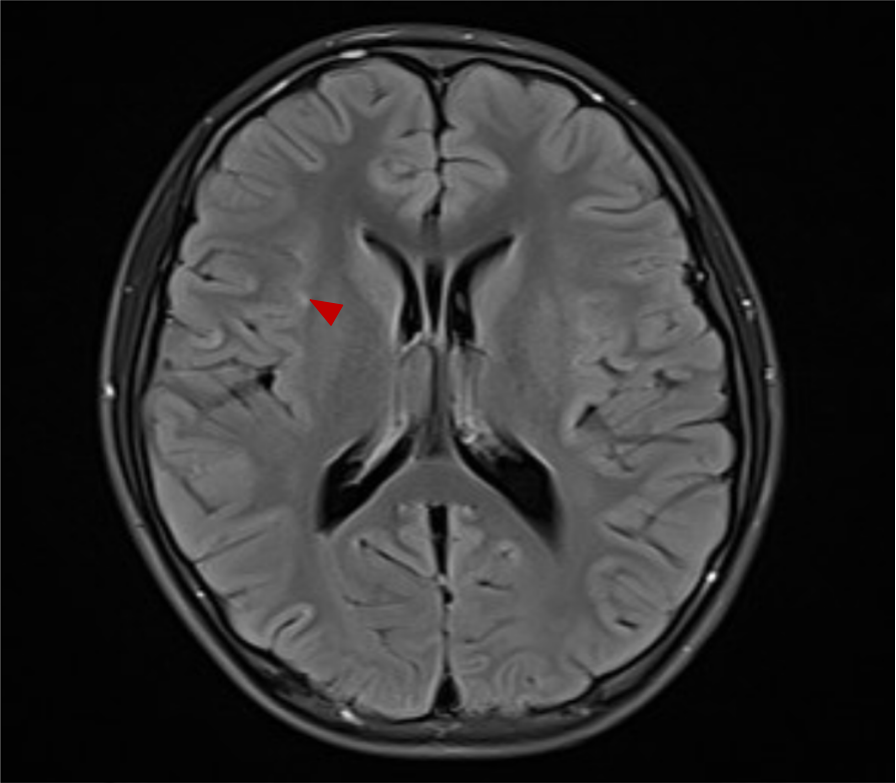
The brain MRI image of the patient. Red arrow showed a few speckled abnormal enhanced signal shadows in the right insular lobe
